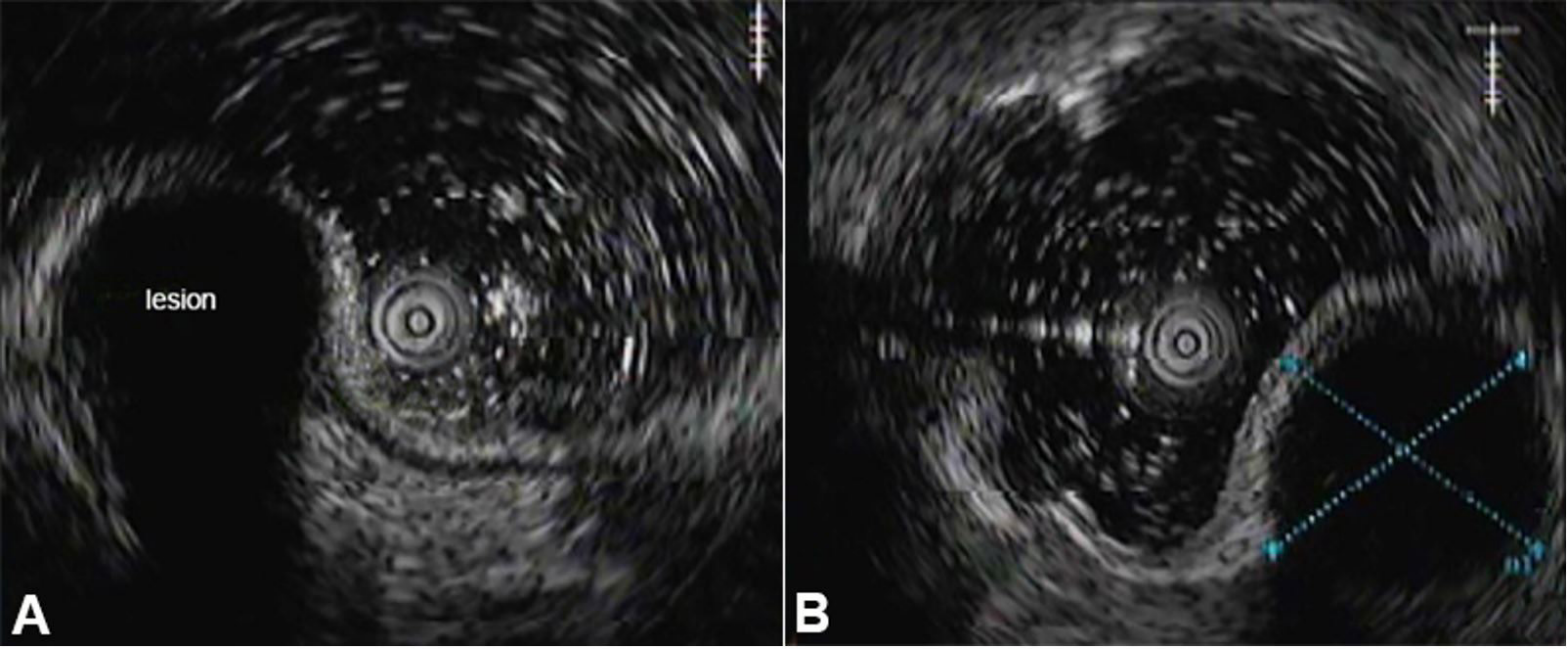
Endoscopic ultrasound of the cecum. Intramural lesion measuring in the cecum’s appendicular orifice. The dependence of the lesion on the submucous (A) or muscularis propria (B) layers was not securely ascertained.
